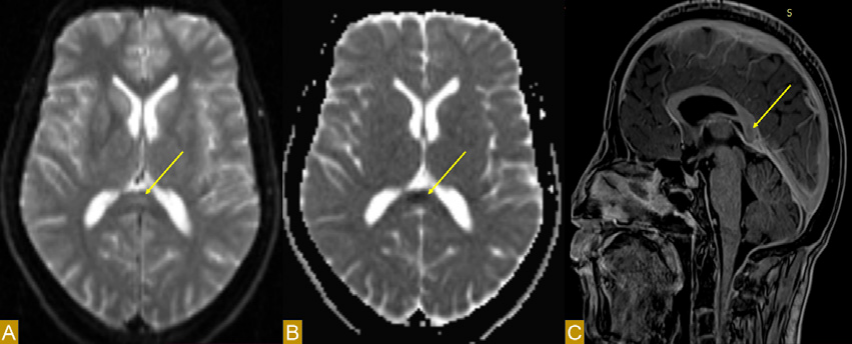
Brain MRI showing a well-defined, midline focal lesion in the splenium of the corpus callosum (arrow), characterized by hyperintensity on the diffusion sequence (A) and a drop in signal on the apparent diffusion coefficient map (B), with no enhancement after gadolinium injection (C).
